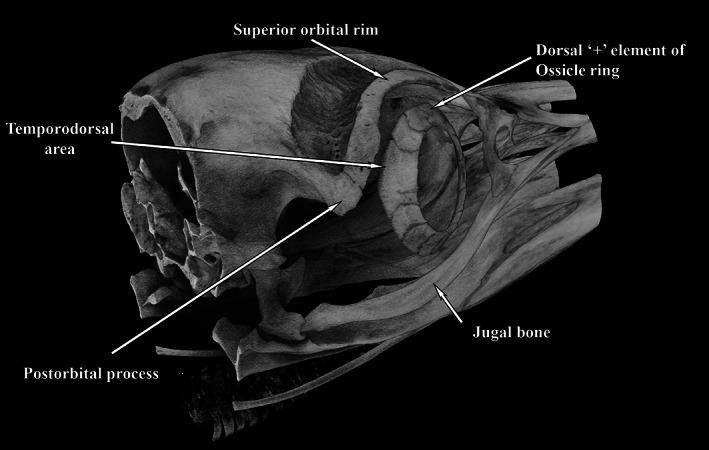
Micro‐CT image of the whole head of an adult gentoo penguin (*Pygoscelis papua*, G1) showing a dorsal ‘+’ element at 12 o'clock. The area between the postorbital process and the jugal bone contains the thick musculus adductor mandibulae externus, whose bulk would protect the globe in that area. The part of the globe least protected by the bony orbit can be seen to be the dorsal and temporo‐dorsal area, where the ring was thickest. Length of head imaged = 80 mm
